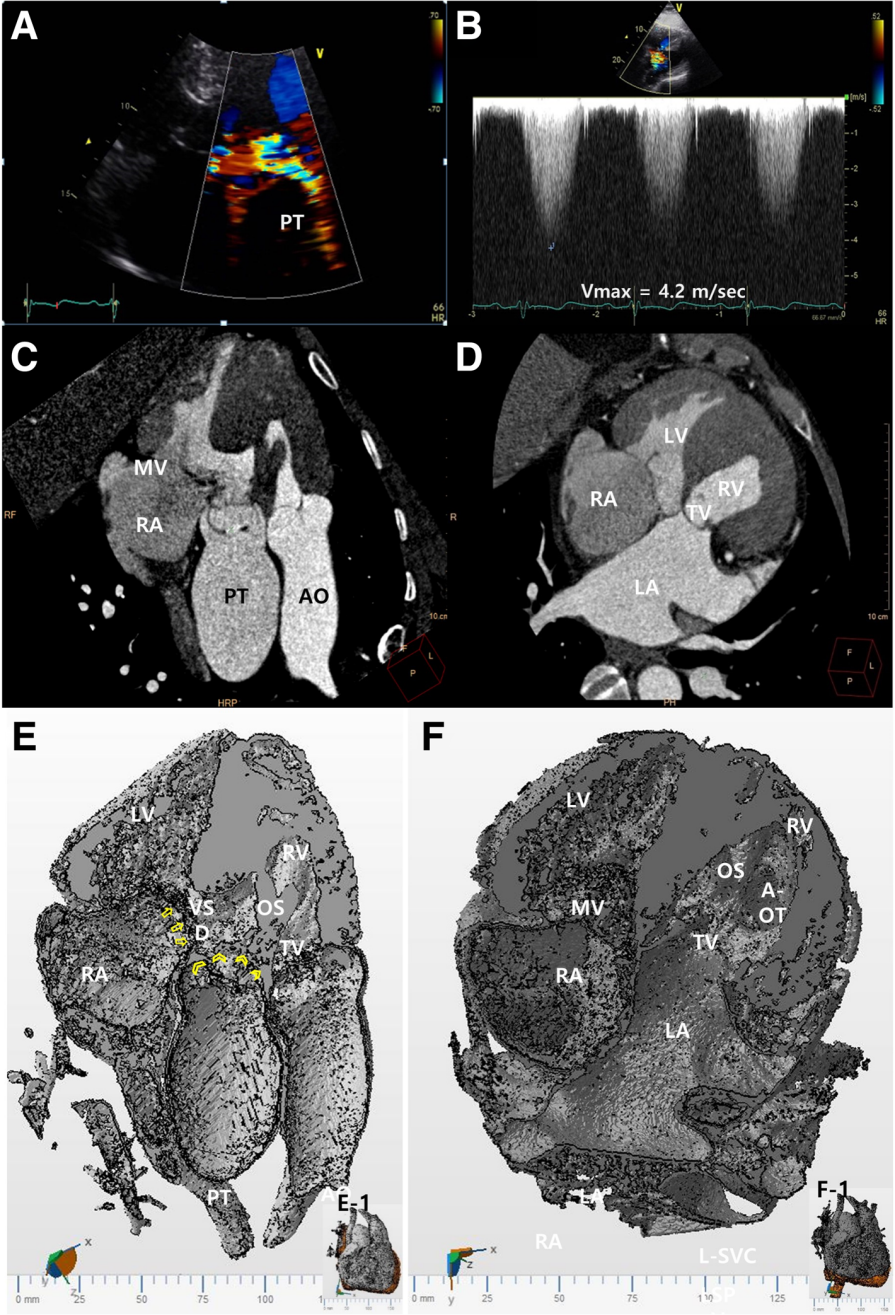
a and b Transthoracic echocardiography with color Doppler detected a severe pulmonic stenosis, with a peak velocity of 4.2 m/second. c Cardiac computed tomography image shows the pulmonic trunk overriding and straddling the ventricular septum. The anterior leaflet of the mitral valve is attached to the ventricular septum near the pulmonary valve. The mitral valve together with the outlet septum of the right ventricle caused the subvalvular pulmonary stenosis. The subaortic part is apparently stenotic in this view. d Cardiac computed tomography image also shows the subvalvular structure of the tricuspid valve connected to the ventricular septum. e A three-dimensional model of the cardiac computed tomography at a plane of (c) (as shown in E-1) shows detailed anatomy of the sub-pulmonary structure and its relation to the ventricular septum. f A three-dimensional model of the cardiac computed tomography at a plane similar to that in (d) (as shown in F-1) shows the widely open aorta outflow tract. The aortic outflow is separated from the stenotic pulmonary outflow tract by a prominent outlet septum. (Supplementary video is available in Additional files 3 and 4). *AO* aorta, *A-OT* aorta outflow tract, *LA* left atrium, *LV* left ventricle, *MV* mitral valve, *OS* outlet septum, *PT* pulmonic trunk, RA right atrium, *RV* right ventricle, *TV* tricuspid valve, *VSD* ventricular septum defect
